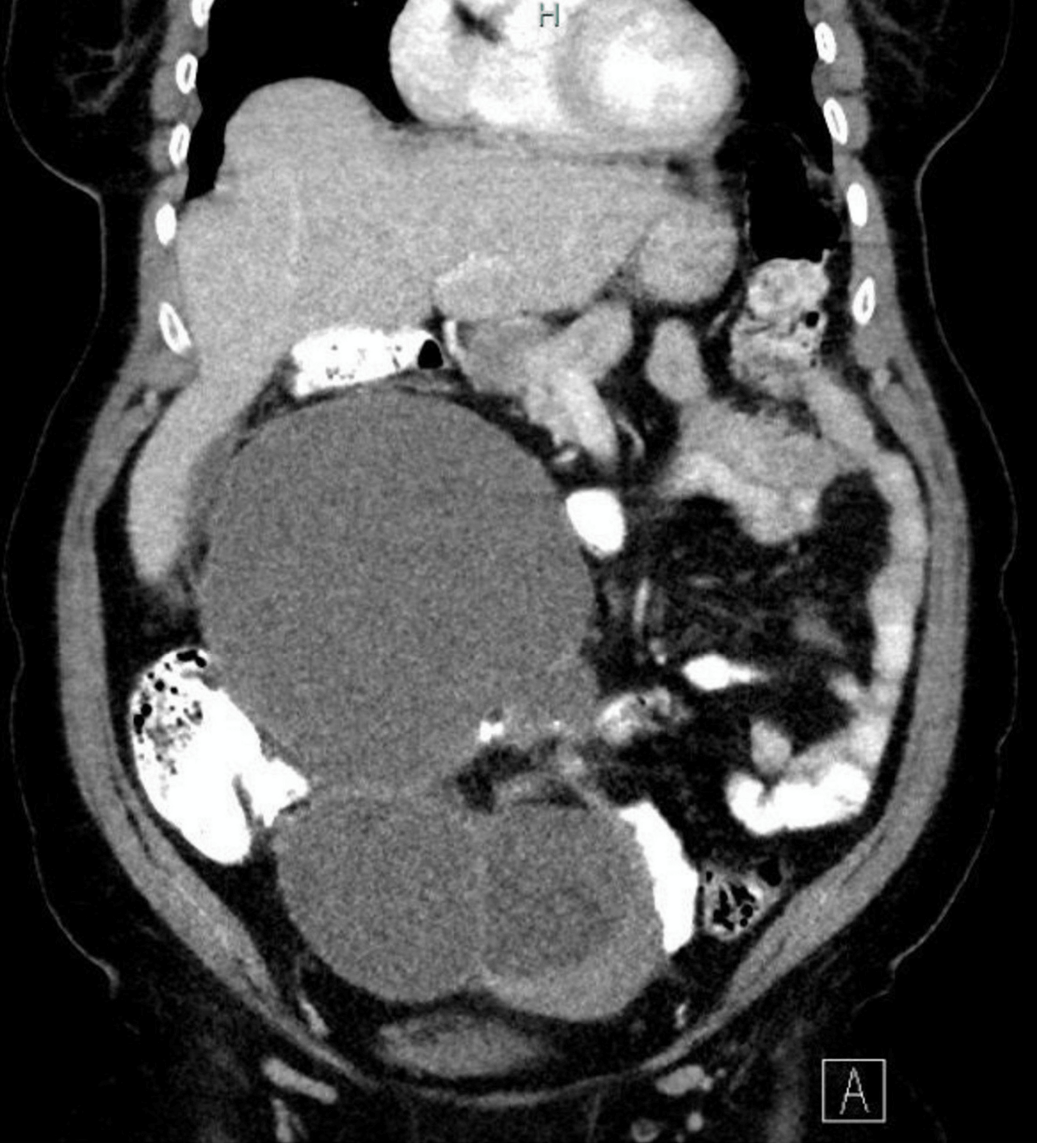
Contrasted axial CT imaging of the abdomen and pelvis Contrasted axial CT imaging of the abdomen and pelvis reveals a large (27 × 13 × 8.5 cm), well-circumscribed, heterogeneous pelvic mass consisting of solid and cystic portions, consistent with a mature teratoma with some degree of malignant transformation. The mass is displacing adjacent bowel loops and compressing the urinary bladder inferiorly. There is an associated soft tissue extension involving the mesoappendix, concerning for local invasion. The liver, kidneys, and upper abdomen are seen and appear uninvolved at current levels.
